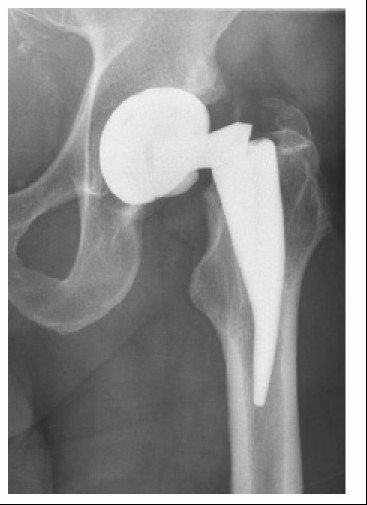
X-ray of a hip with a failed neck.
